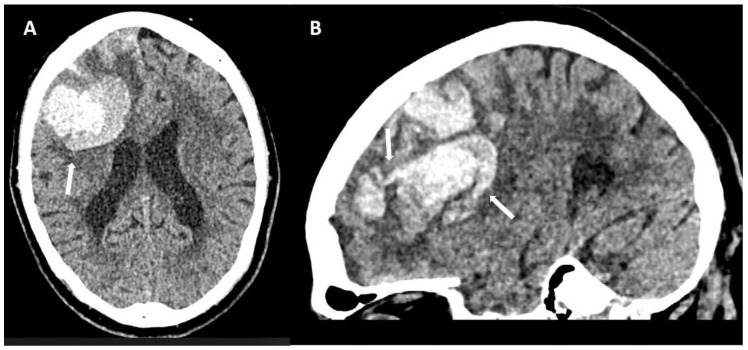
Demonstrating the need for 3D reconstruction. No finger-like projection can be spotted on non-enhanced CT (100 kV, 394 mAs, slice thickness 1 mm) in the axial plane, only a lobulation ((A), arrow). The sagittal reconstruction demonstrates at least two finger-like projections ((B), arrows).
